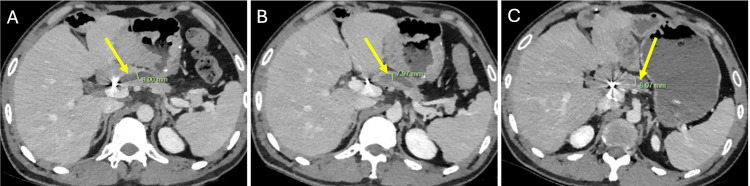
CT images demonstrating persistent dilatation of the remnant main pancreatic duct from March 2021 to April 2022 Axial CT imaging with portal venous phase contrast enhancement demonstrating persistent dilatation of the remnant main pancreatic duct (arrow) at approximately 8 mm in (A) March 2021, (B) October 2021, and (C) April 2022. CT, computed tomography.
